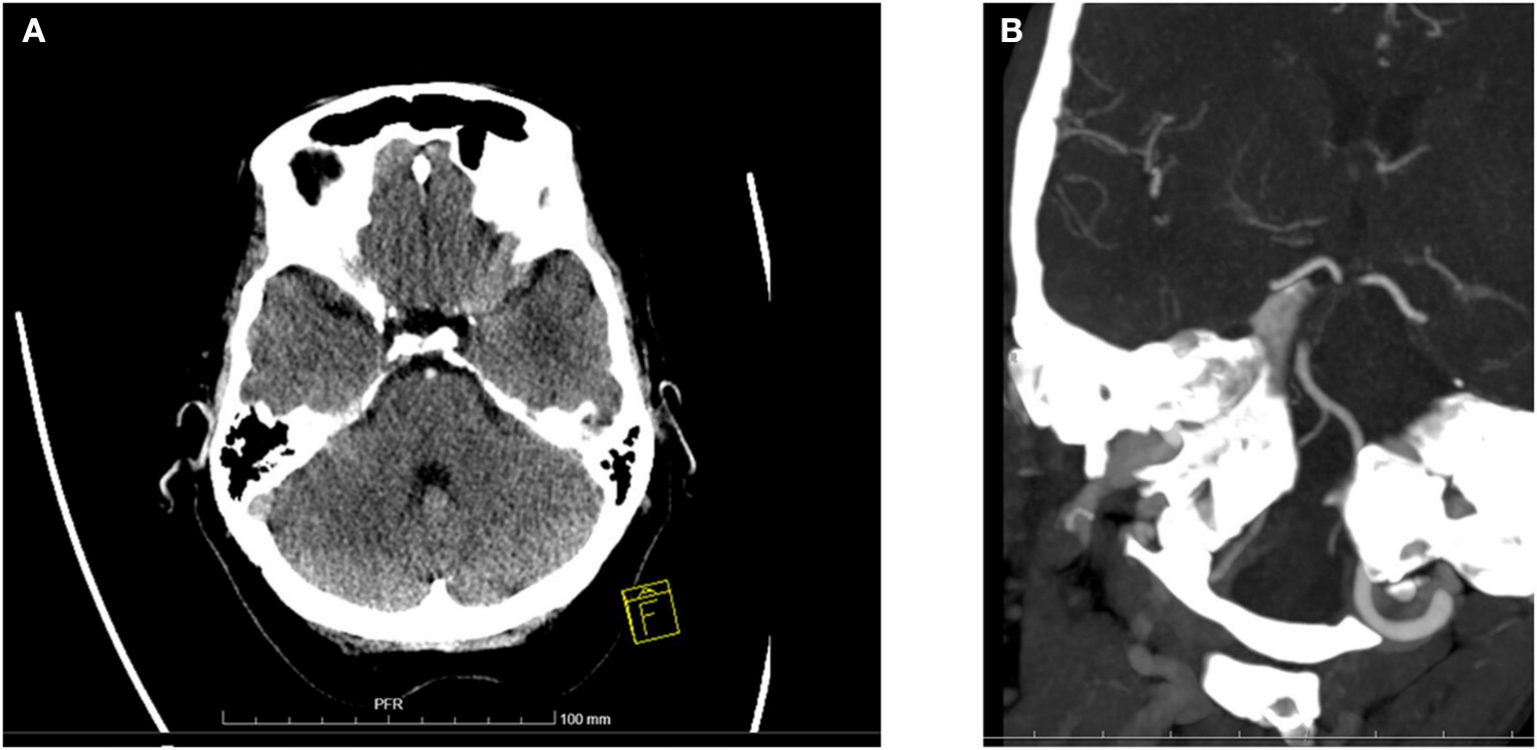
Baseline ischemic changes and basilar artery occlusion in computer tomography. (A) A 69-year-old woman was comatose on admission (GCS 4) and diagnosed with basilar artery occlusion. This baseline CT scan shows extensive ischemic changes in both cerebellar hemispheres and bilaterally in pons. Overall pc-ASPECTS was 5. A dense basilar artery is also seen. Patient received IVT but died on day 3 of hospitalization. (B) A 65-year-old woman was comatose on admission (GCS 6). Baseline CTA shows long BAO over mid- and caudal BA. IVT was administered and subsequent mechanical thrombectomy was performed with successful recanalization. Patient recovered with a 3-month mRS of 1.
